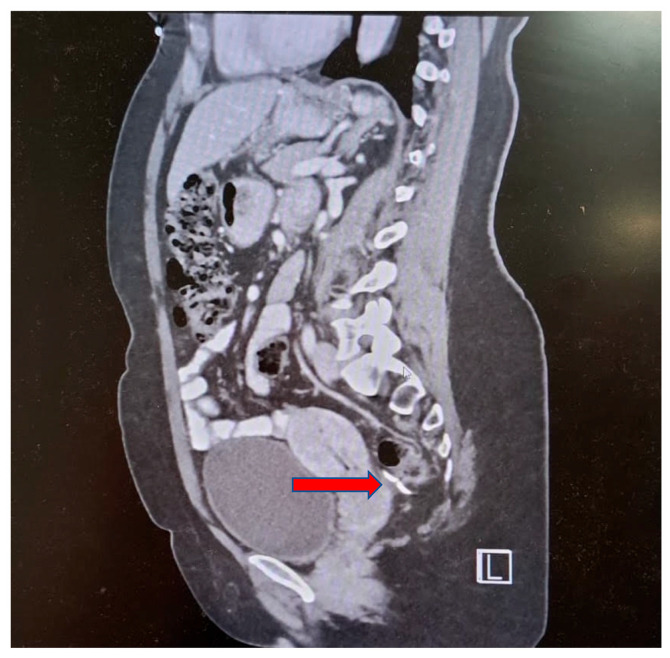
Sagittal image of an abdominal CT scan showing the IUD arms embedded in the rectal wall.
